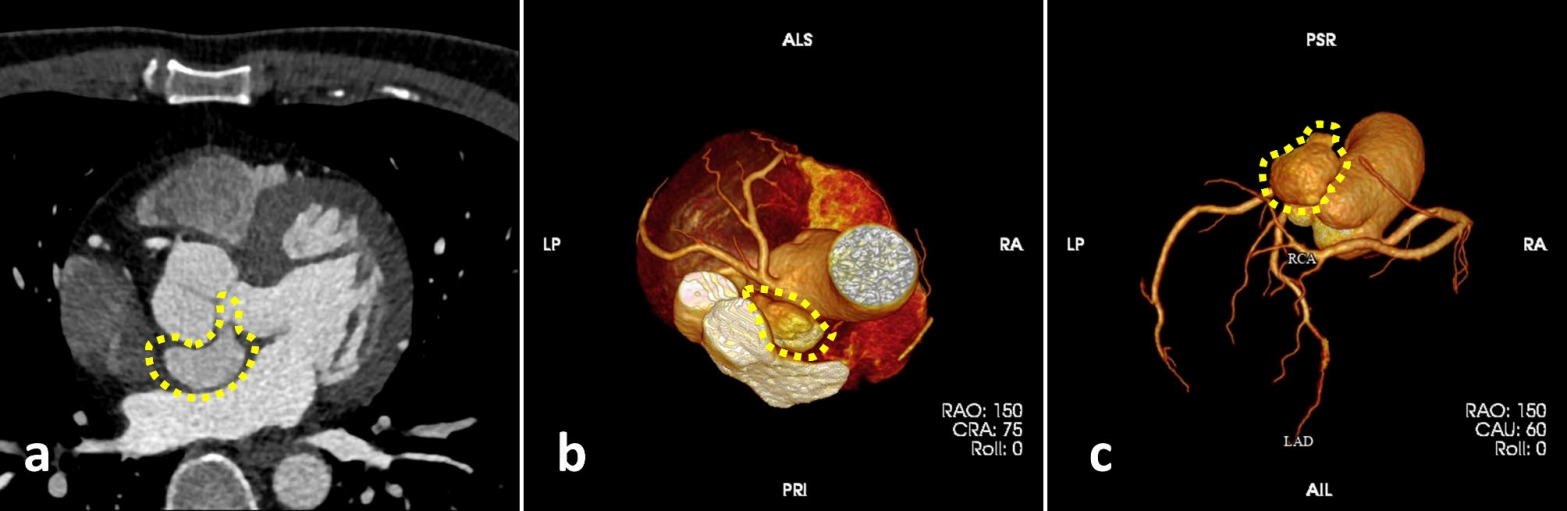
Contrast-enhanced CT image revealing the morphology of pseudoaneurysm. The yellow dotted line indicates the P-MAIVF.
